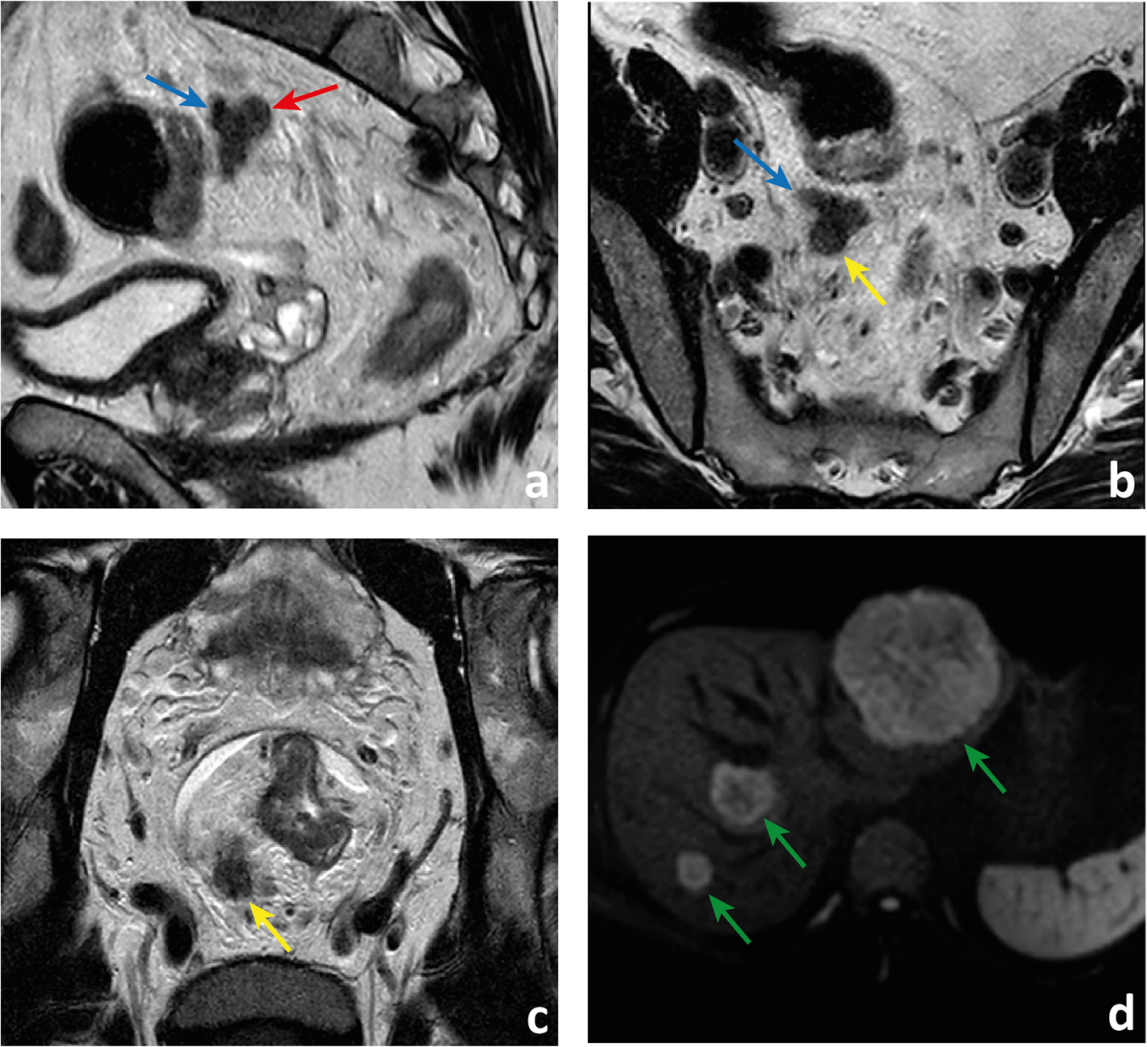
A patient with an mrT3d MRF+ high rectal cancer also presents with a very irregular hypointense lesion in the high mesorectum (red arrow in a) that appears to both interrupt the course of a vein suggesting mrENTD (blue arrow in a) and to grow within it suggesting discontinuous mrEMVI (blue arrow in b). It is also in continuity with a round, irregular and heterogeneous structure appearing to be a positive lymph node with extracapsular extension (yellow arrows in b and c). The patient presented with synchronous metastatic liver disease, visible on b900 DWI (green arrows in d)
